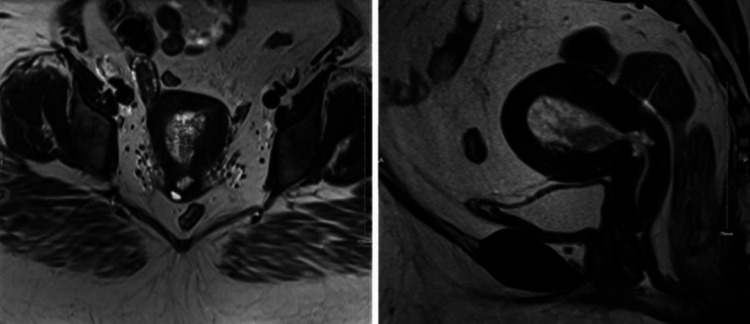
Pelvic MRI of a medically inoperable endometrial cancer patient. T2 pelvic MRI axial (left) and sagittal (right) images of a medically inoperable endometrial cancer in a morbidly obese patient. Due to the concern for cervix involvement noted on the sagittal MRI, this patient was treated with external beam radiation therapy followed by a brachytherapy boost using a Y-tandem applicator.
